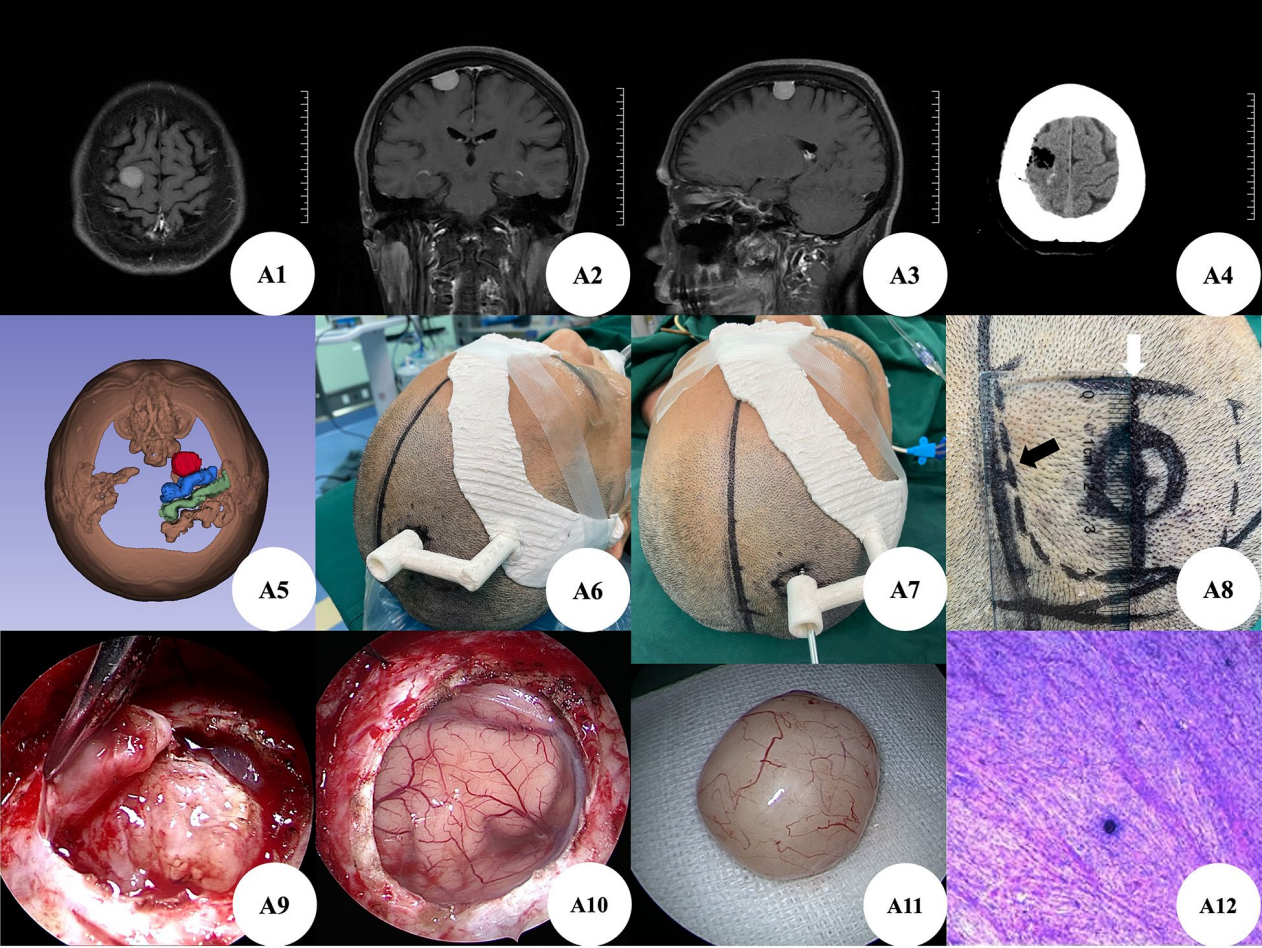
(A1–A3): Brain MRI enhancement showed right frontal lobe space occupying lesions with uniform enhancement and clear boundary; (A4): Postoperative brain CT showed that the intracranial lesions were removed completely; (A5): Relationship between intracranial lesions and brain tissue of anterior central gyrus and posterior central gyrus reconstructed by 3D-Slicer; (A6–A7): 3D printing positioning guide; (A8): According to the surgical incision design after 3D positioning guide plate positioning, the circle is the body surface projection of intracranial lesions, the solid line straight incision is the improved small incision, the ruler shows that the surgical incision is about 4 cm, and the dotted line “U” incision is the conventional surgical incision; (A9): The lesion can be seen by cutting the dura mater under transcranial neuroendoscopic; (A10): The lesion was removed cleanly without obvious side injury to the surrounding tissue under transcranial neuroendoscopic; (A11): Completely resected lesions; (A12): The postoperative pathological examination.
